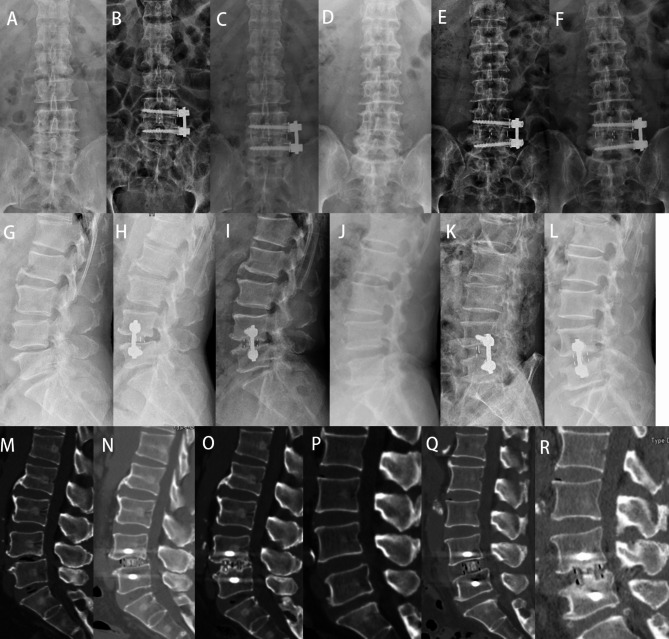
A patient of L4 DLS underwent OLIF-AF. (A, B, C) A-P X-ray preoperatively, 1 day and 24 months postoperatively; (G, H, I) lateral X-ray preoperatively, 1 day and 24 months postoperatively; (M, N, O) sagittal CT preoperatively, 1 day and 24 months postoperatively. CS occurred, no fixation loosening was observed and fusion was accomplished. A patient of L4-5 DLSS underwent OLIF-AF. (D, E, F) A-P X-ray preoperatively, 1 day and 24 months postoperatively; (J, K, L) lateral X-ray preoperatively, 1 day and 24 months postoperatively; (P, Q, R) sagittal CT preoperatively, 1 day and 24 months postoperatively. DH slightly deceased and CS did not occur, no fixation loosening was observed and fusion were all accomplished in both cases
